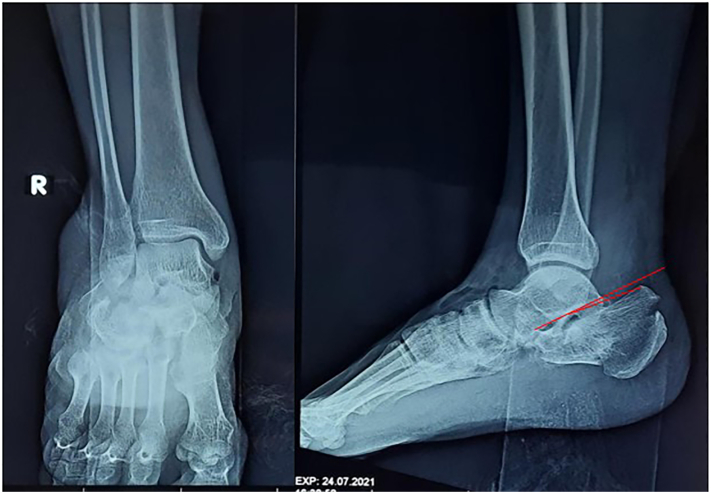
X-Ray pre-operative management with AP/Lateral view of left foot, with Gissane angle: 158° and Bohler angle: 12° (red line). (For interpretation of the references to colour in this figure legend, the reader is referred to the web version of this article.)
